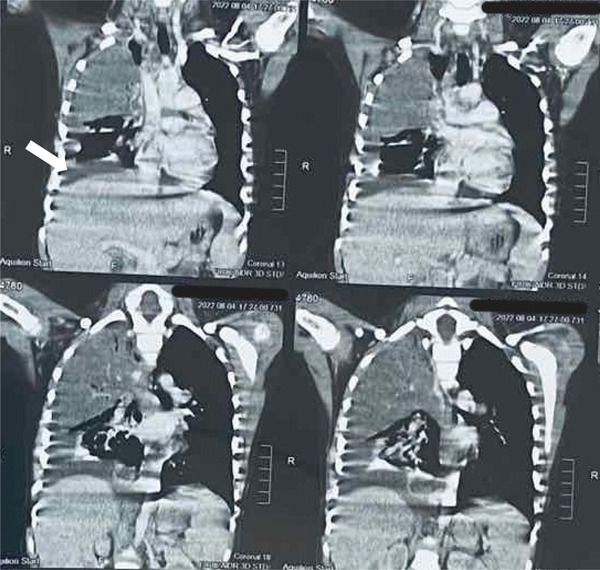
CT chest coronal plane showing right‐sided empyema (white arrow).
